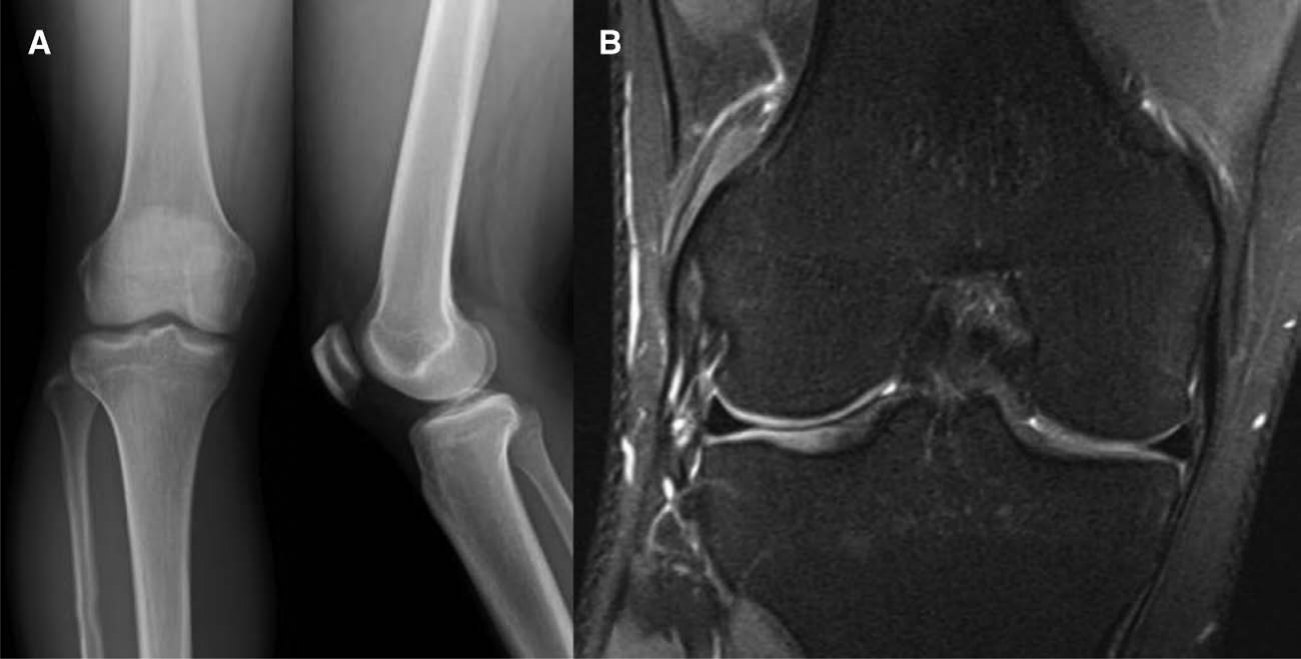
(a) Plain knee radiograph before surgery (b) MRI coronal image: there are no abnormal findings on either the radiograph or the MRI. MRI = magnetic resonance imaging.
